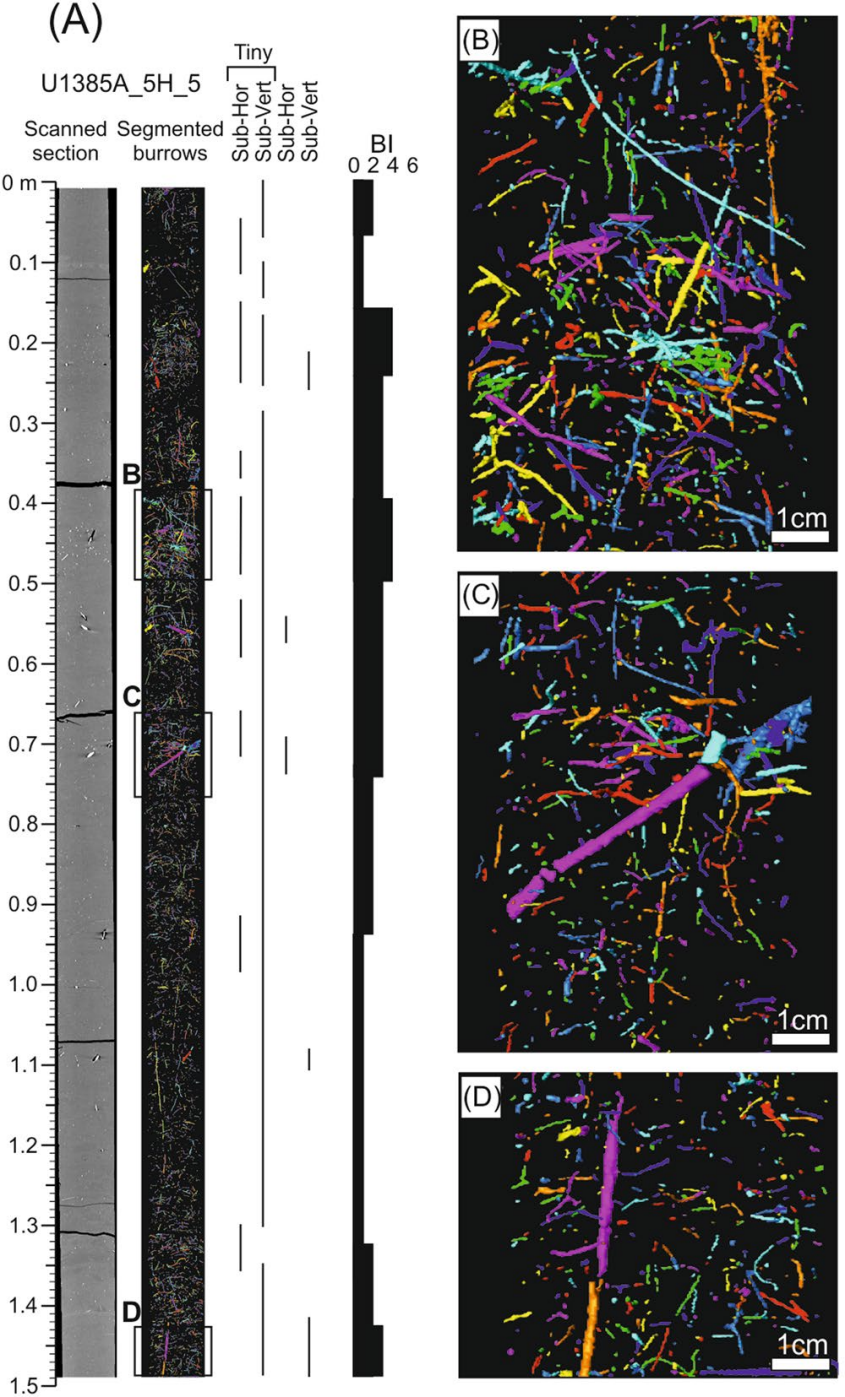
Ichnological content and Bioturbation Index (BI) based on segmented burrows by CT processing from core section U1385A_5H_5.
